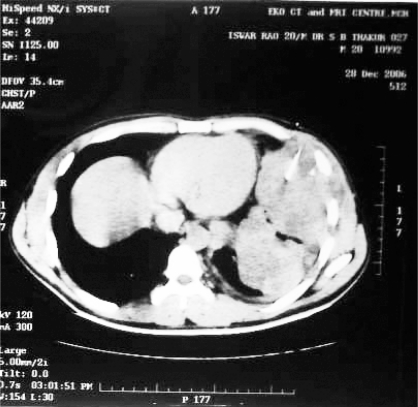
Computed tomography of the same lesion. A CT guided FNAC was taken from the mass
